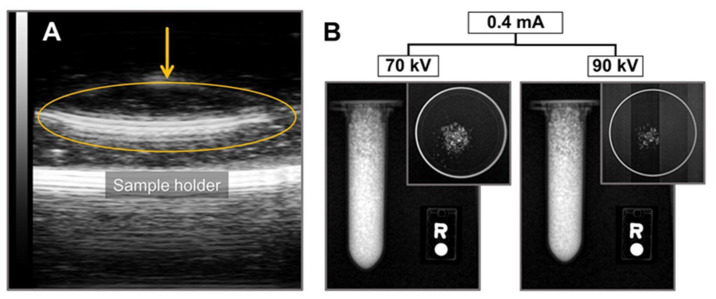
(A) Ultrasound imaging showing *part*-CaF_2_ suspended in water (orange arrow). (B) X-ray image showing a 2 mL Eppendorf tube filled with *part*-CaF_2_ and a top view of powder dispersed into a petri dish subjected to different potential and the same current intensity.
